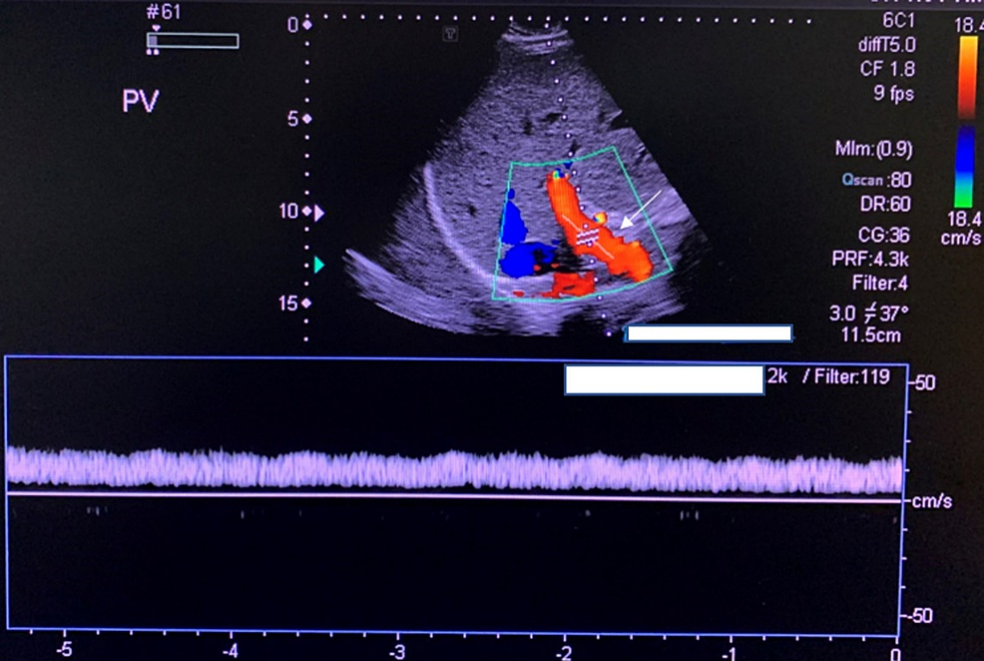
Ultrasound with doppler of the portal vein. The arrow points to the portal vein which demonstrated normal flow and direction of blood on Doppler.
